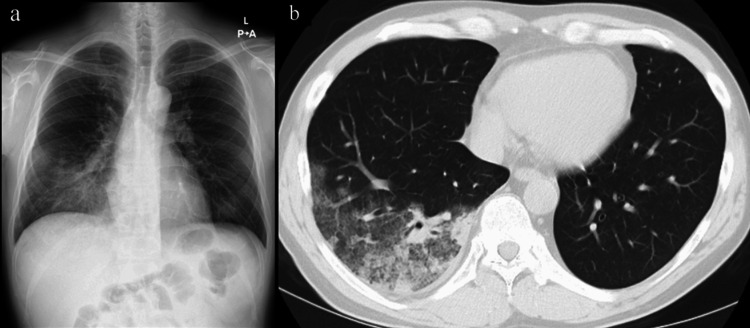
Chest x-ray and chest CT of the present case on admission to our hospital. (a) The frontal chest radiograph shows a patchy consolidation in the lower lobe of the right lung; (b) the axial chest CT (lung window) shows a multifocal consolidation with air bronchograms in the right lower lobe, accompanied by ground-glass opacities.
